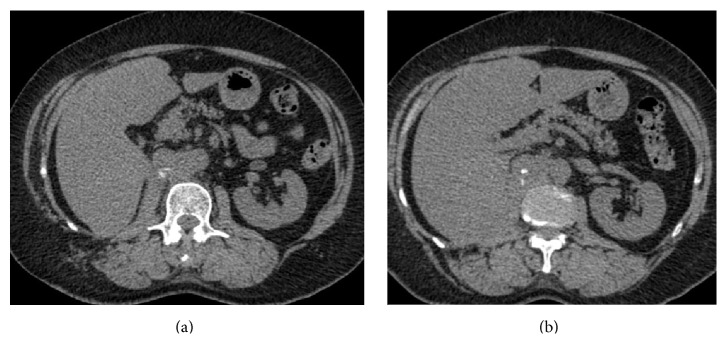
CT scan performed after 4 cycles of chemotherapy. Axial nonenhanced CT scan showing (a) increase in size of the interaortocaval lymph node now measuring 2.5 cm indistinguishable from the aorta and the vena cava; (b) increase in retrohepatic tissue thickening along the vena cava.
